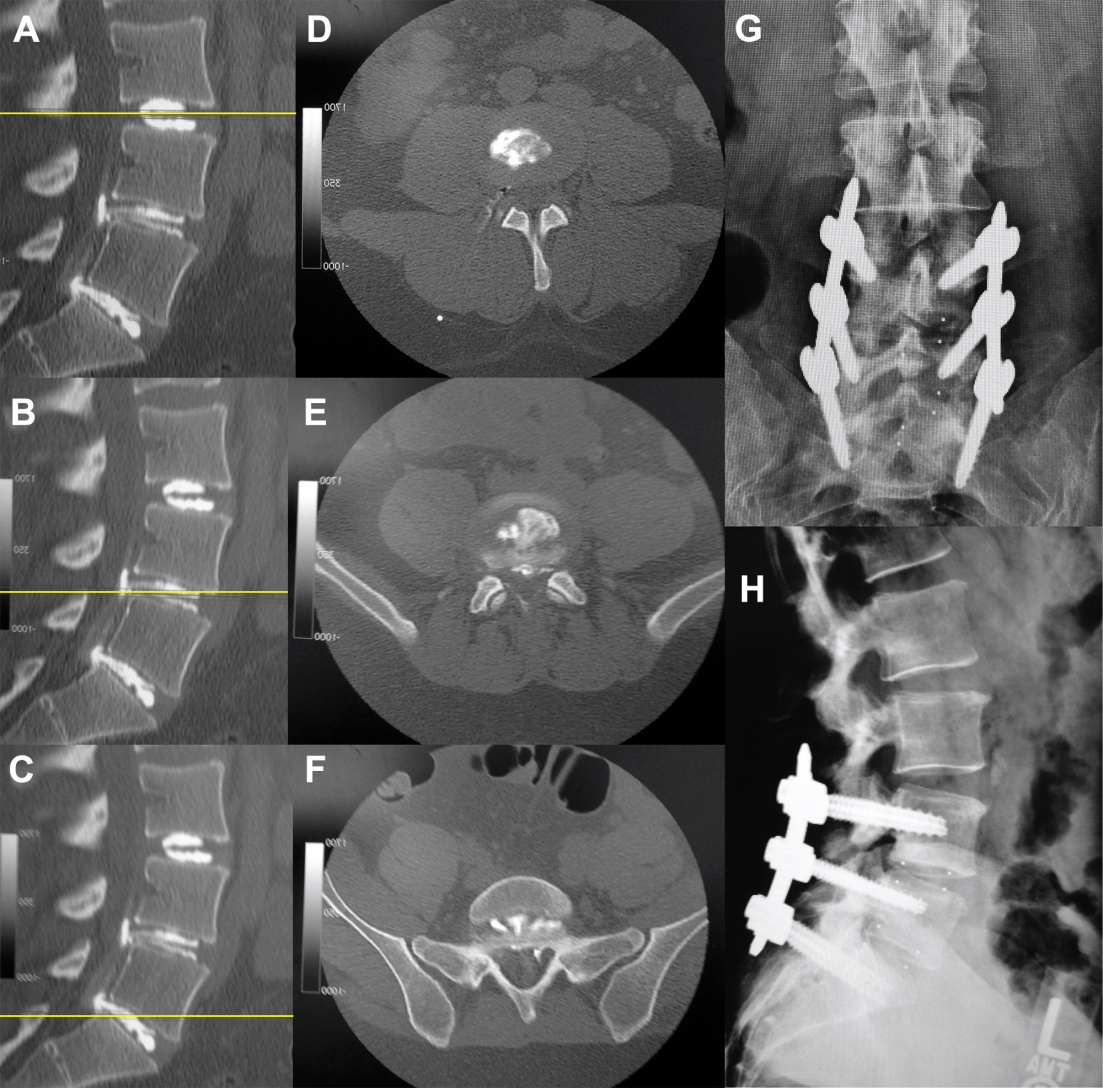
Post-discography CT (A-F) and post-surgical sagittal images (G, H) of a patient with two levels of disc disruption. Sagittal (A-C) and axial (D-F) CT views show morphologically intact discs at L3-4 (A, D) withradio-opaque dye contained within the nucleus pulposus, and structurally violated disc at L4-5(B, E) and L5-S1 (C, F) with dye egression into the spinal canal. Two-level interbody fusion withinstrumentation at L4-5 and L5-S1 successfully and completely resolved the patient’s low backpain (G, coronal view; H, sagittal view).
